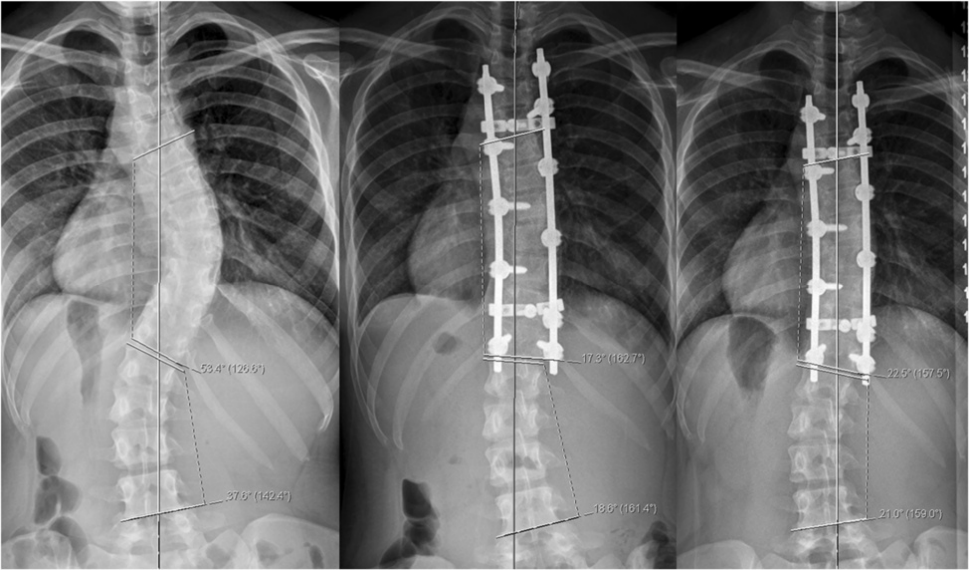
Double structural AIS (*MHHN curve type II*) in 14.5y/♀ treated with *selective thoracic fusion* (65 % correction) with f/u of 3.5 years showing pre-op, immediate post-op and 3.5 years follow-up radiographs
